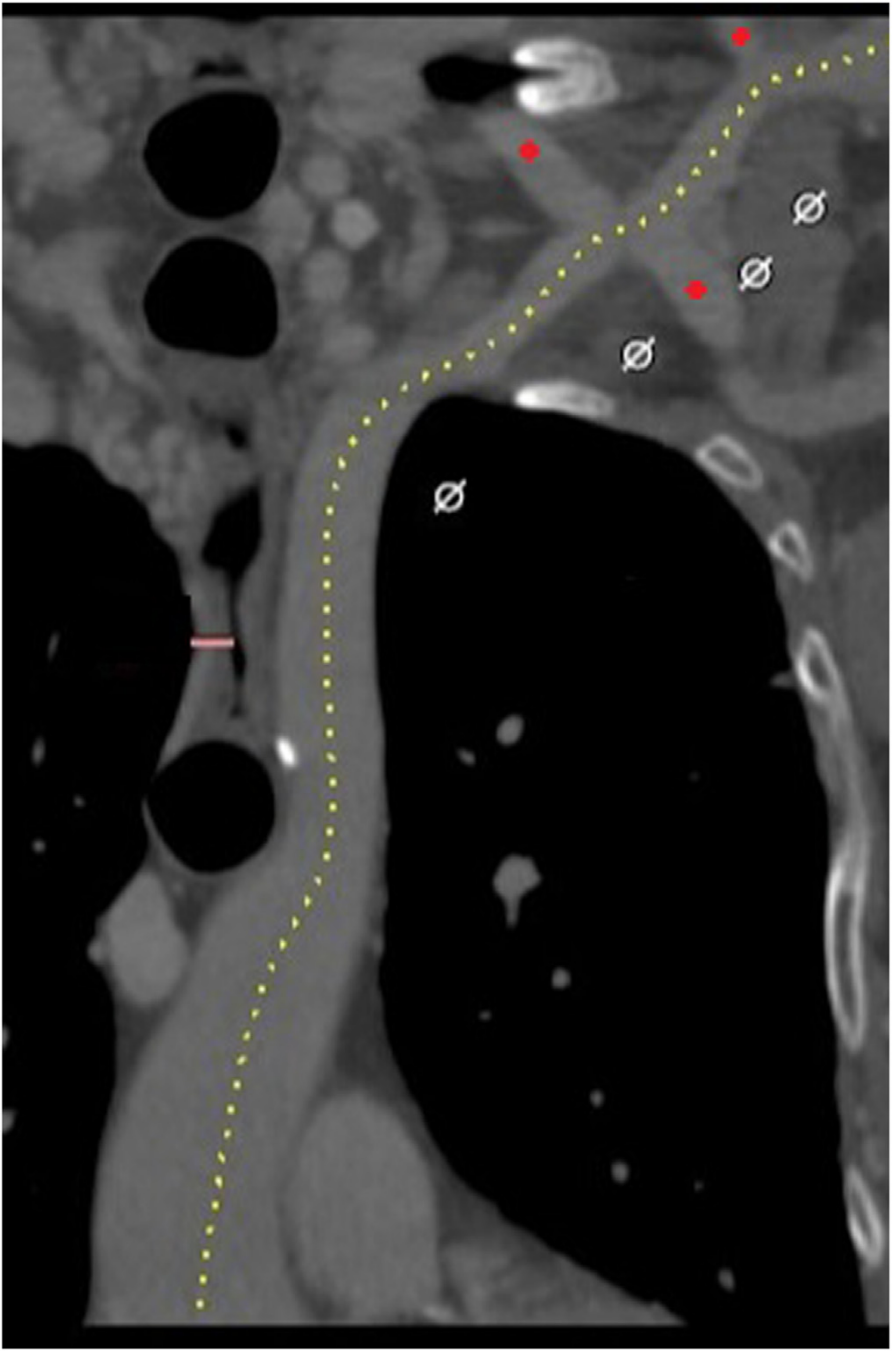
A CTangiogram of the left subclavian artery. Large collateral branches that bypass the coarcted aorta originate from the left subclavian artery and are marked with a red button. A left carotid, axillary artery, or subclavian artery for transcatheter aortic valve replacement was not selected in this case to not disrupt these collateral branches. CT, computed tomography.
